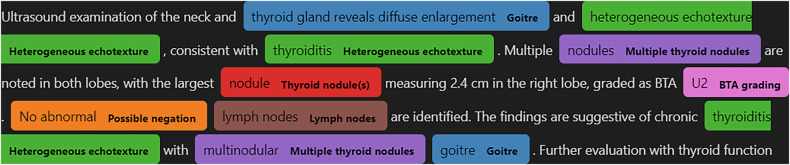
A synthetic neck and thyroid ultrasound report, highlighted using a rule-based radiology data extraction tool. This example was correctly classified as positive for goitre (blue), altered (heterogeneous) thyroid echotexture (green), multiple thyroid nodules (purple), and lymph node examination (brown). The phrase ‘no abnormal’ was matched as a possible negation modifier to the term ‘lymph nodes’
